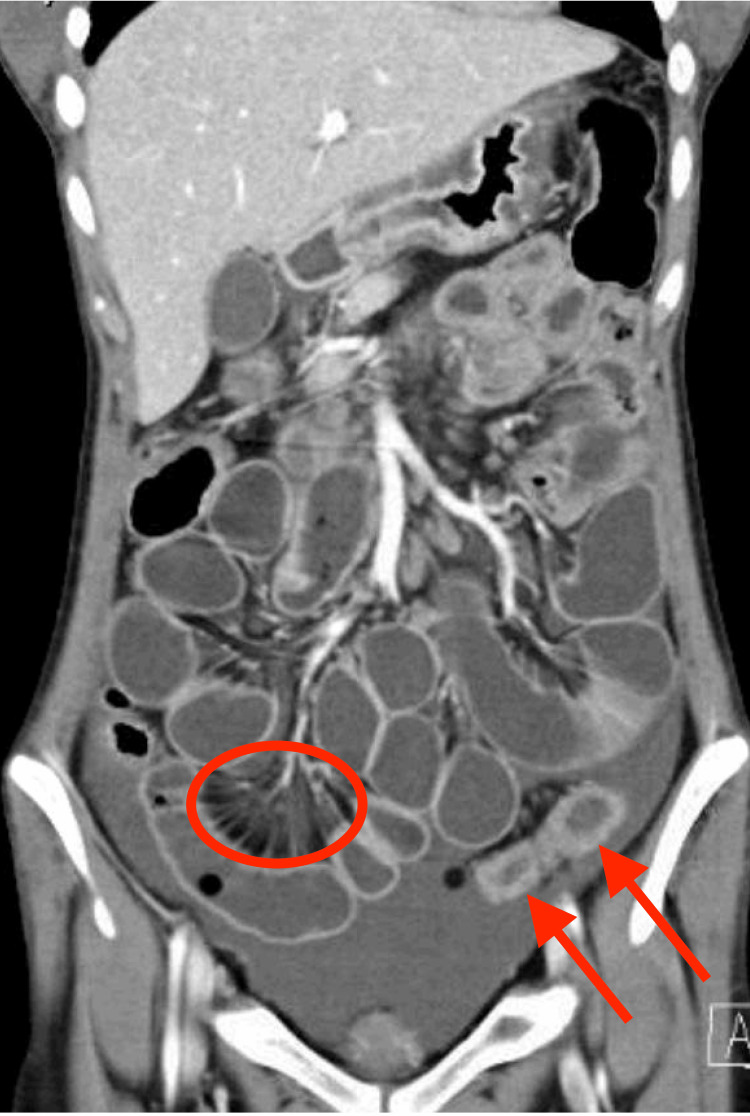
Sagittal contrast enhanced CT scan of the abdomen and pelvic showing circumferential small bowl wall thickening and edema “arrows” with prominent engorgement of mesenteric vessels (comb sign “circle”).
